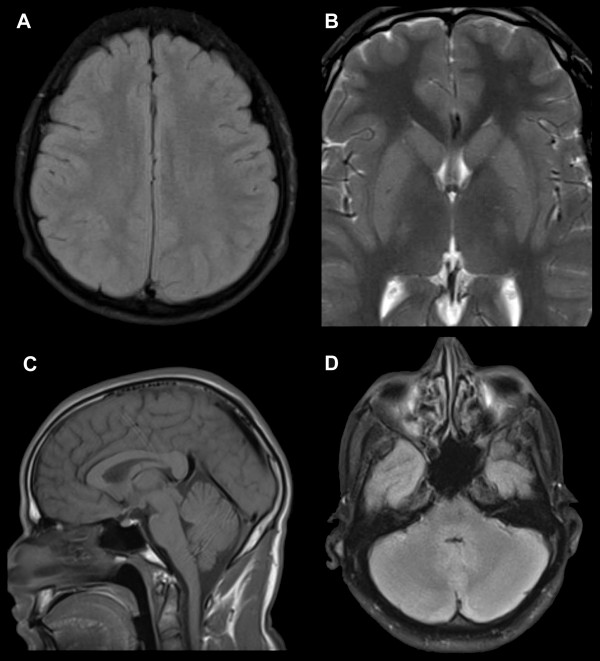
Examples of MRIs from 4 patients with severe malaria. A diffuse moderate supratentorial swelling on FLAIR with obliteration of sulcal pattern, B bilateral swollen striatum on T2 with mildly increased signal intensity and blurred borders, C diffuse mild supratentorial and marked poster fossa swelling on T1 and D marked posterior fossa swelling and mild signal increase on FLAIR.
